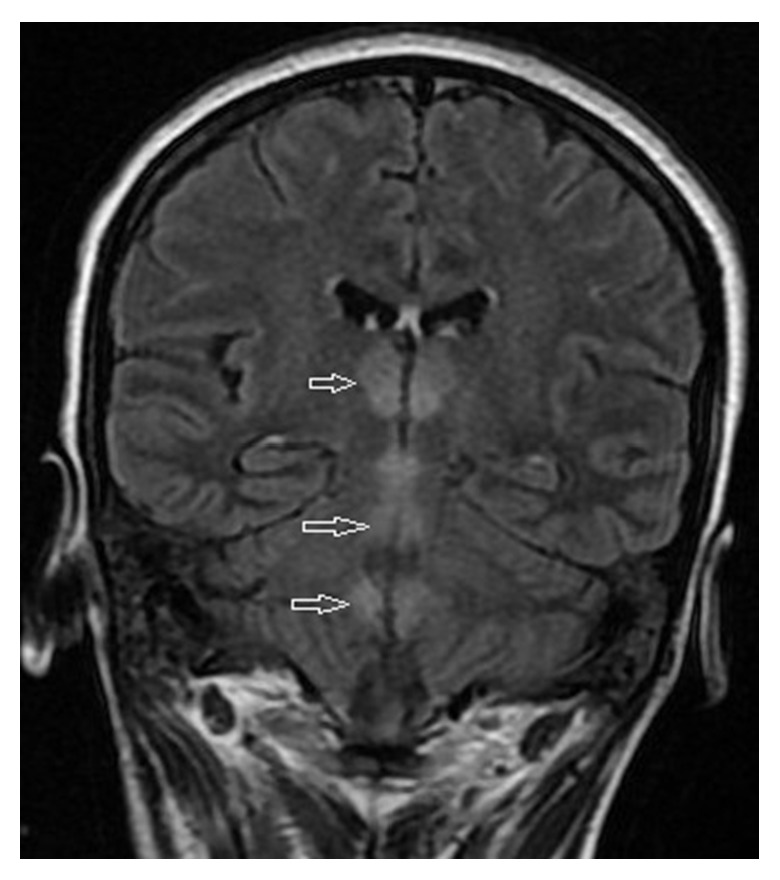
Magnetic resonance imaging of brain showed symmetrical hypersignal intensity at bilateral posterior thalamus and periaqueductal area on fluid-attenuated inversion recovery series.
